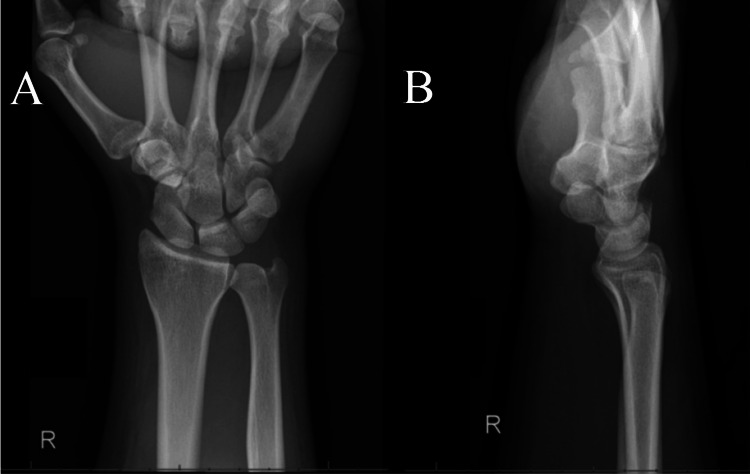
Anterior/posterior radiographs of the right wrist five days after reduction. (A and B) Anterior/posterior and lateral radiograph views of the right wrist, respectively, five days after reduction. These images were stable from prior and revealed no occurrence of dislocation.
